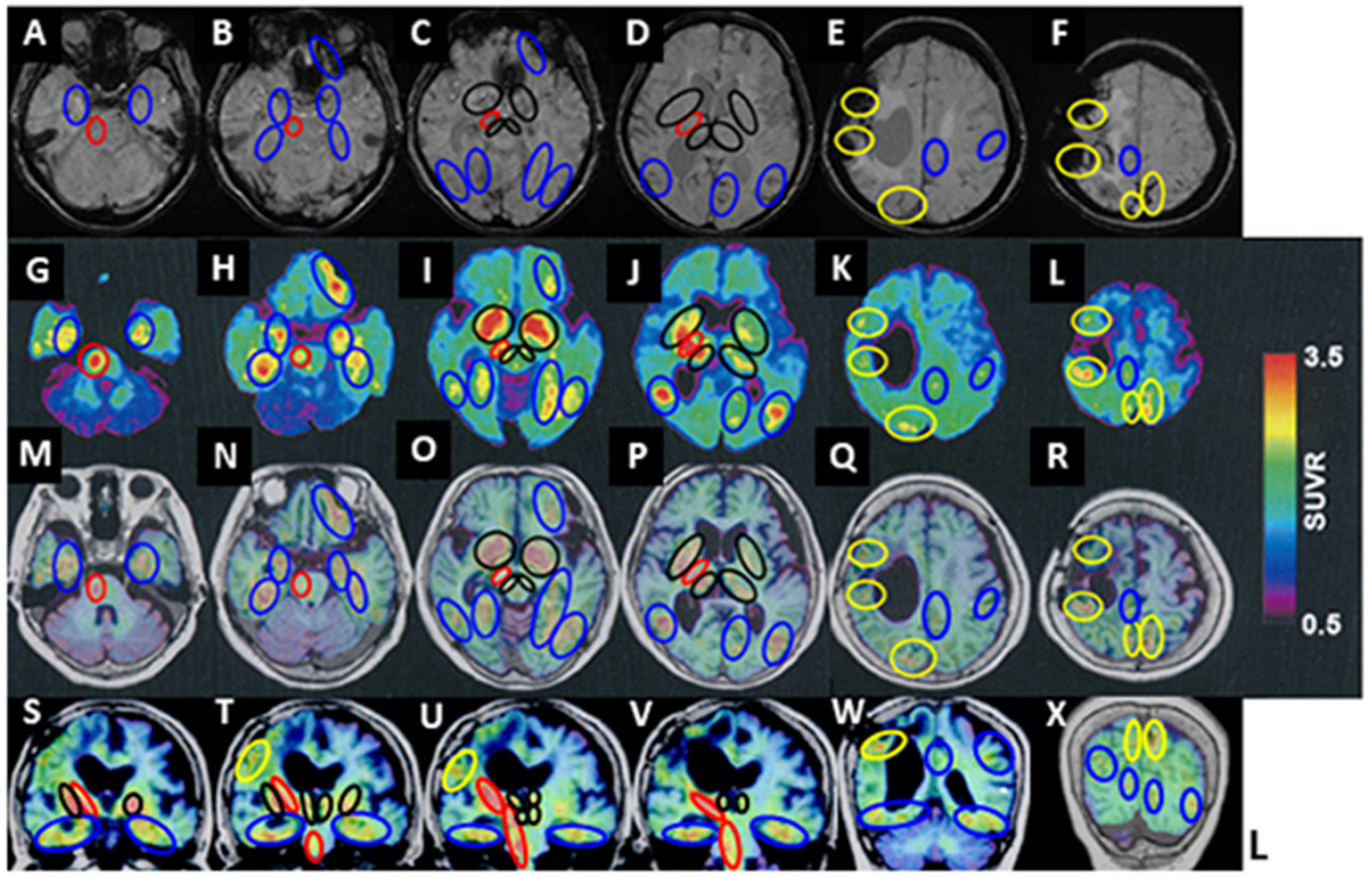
^18^F-THK5351 imaging and MRI of the patient's brain. (A–F) Susceptibility-weighted MRI. (G–L)
